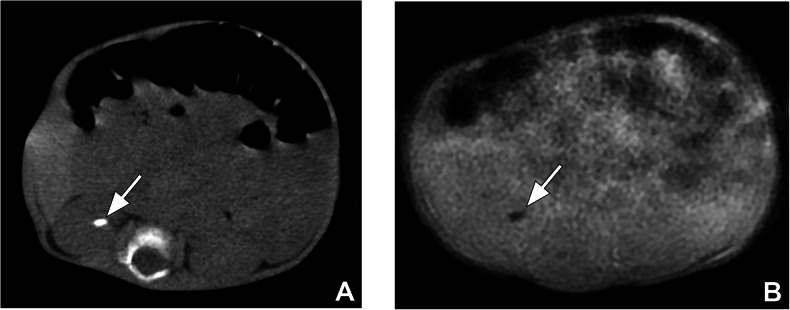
A 1-year-old girl with a right renal stone. A Axial non-enhanced CT and (B) ZTE-MR images show renal stone on the right side (arrows)
